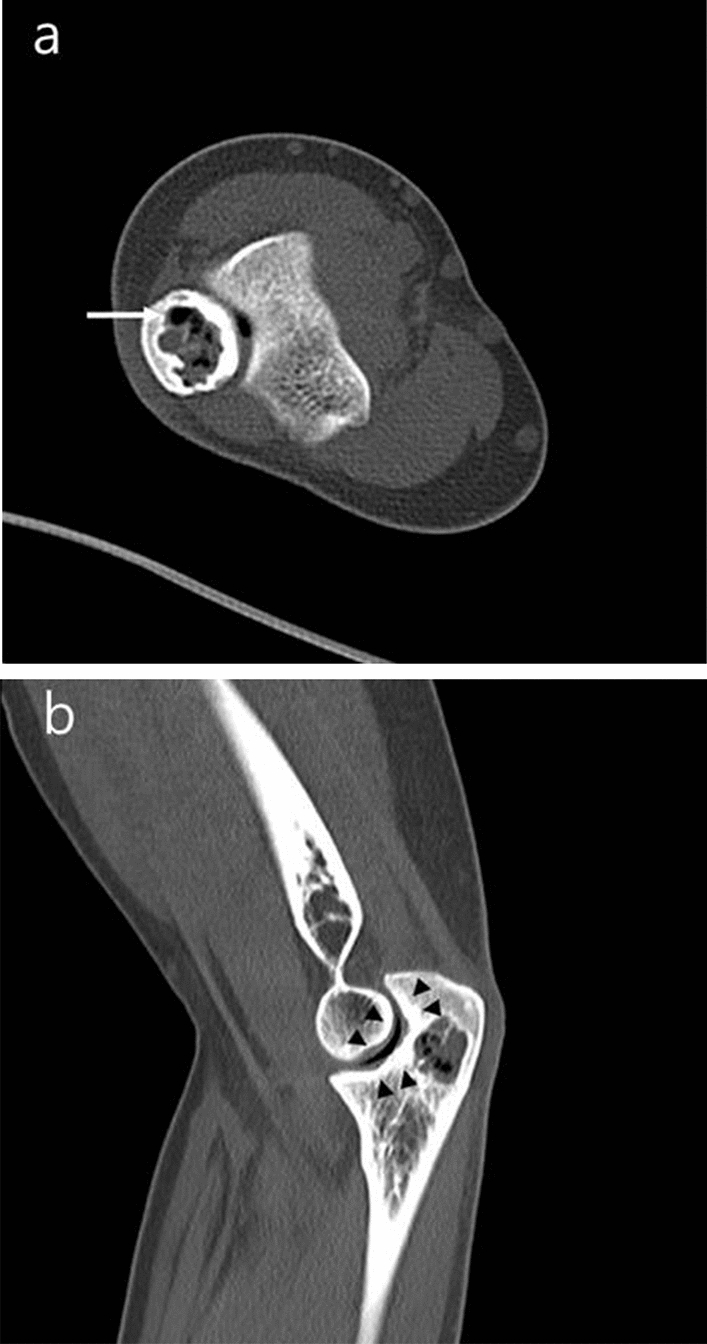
Axial (a) and sagittal (b) CT scan revealed intralesional gas (white arrow) without evidence of underlying osteoid or chondroid mineralization. Vacuum phenomenon was observed in ulnotrochlear joint with subcortical sclerosis and articular surface dimpling (black arrowheads), but there is no continuation between joint and osteolytic bone lesion
